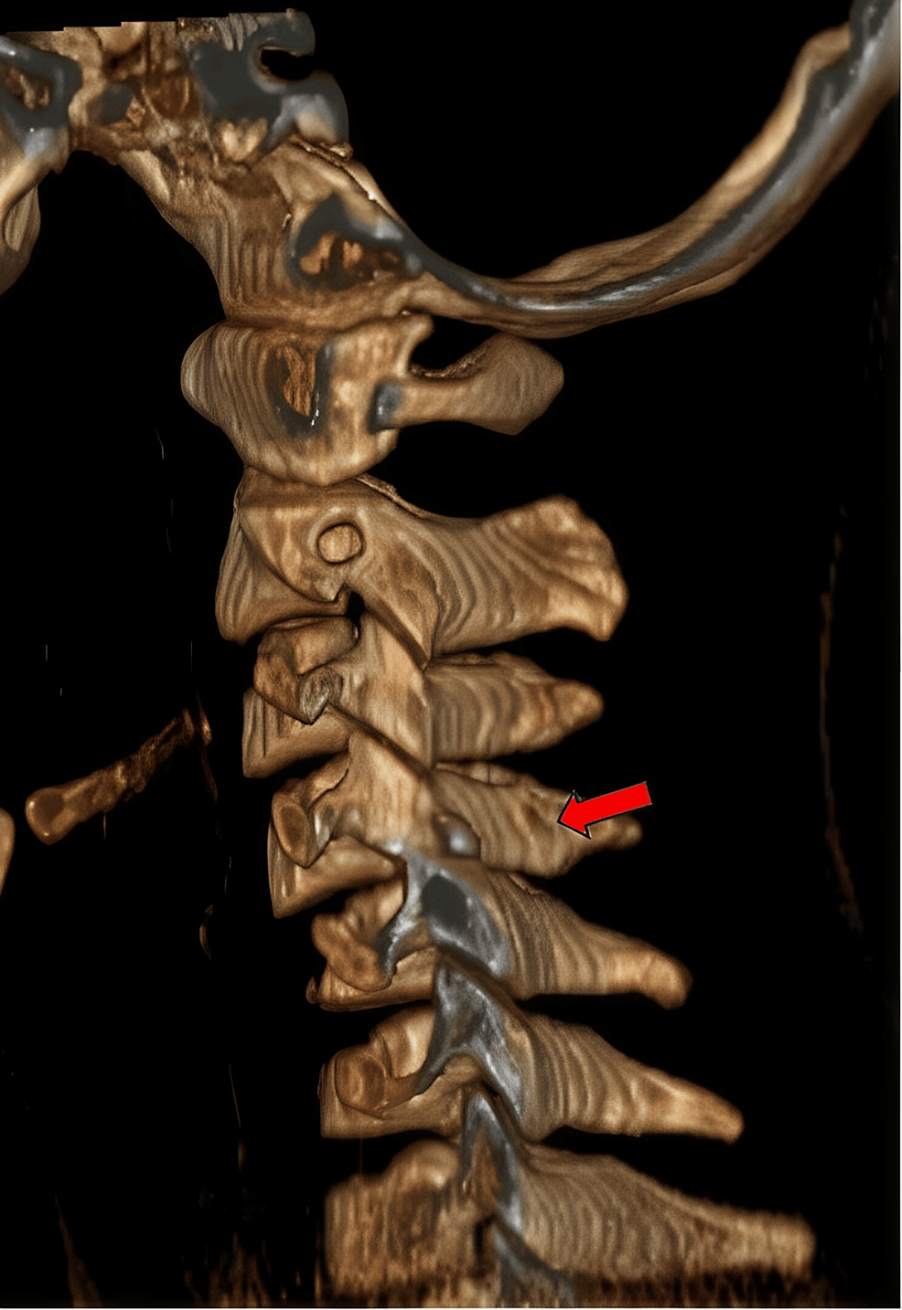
3D reconstruction image of CT scan with red arrow showing bilateral facet dislocation of C4/C5. 3D: three-dimensional; CT: computed tomography
